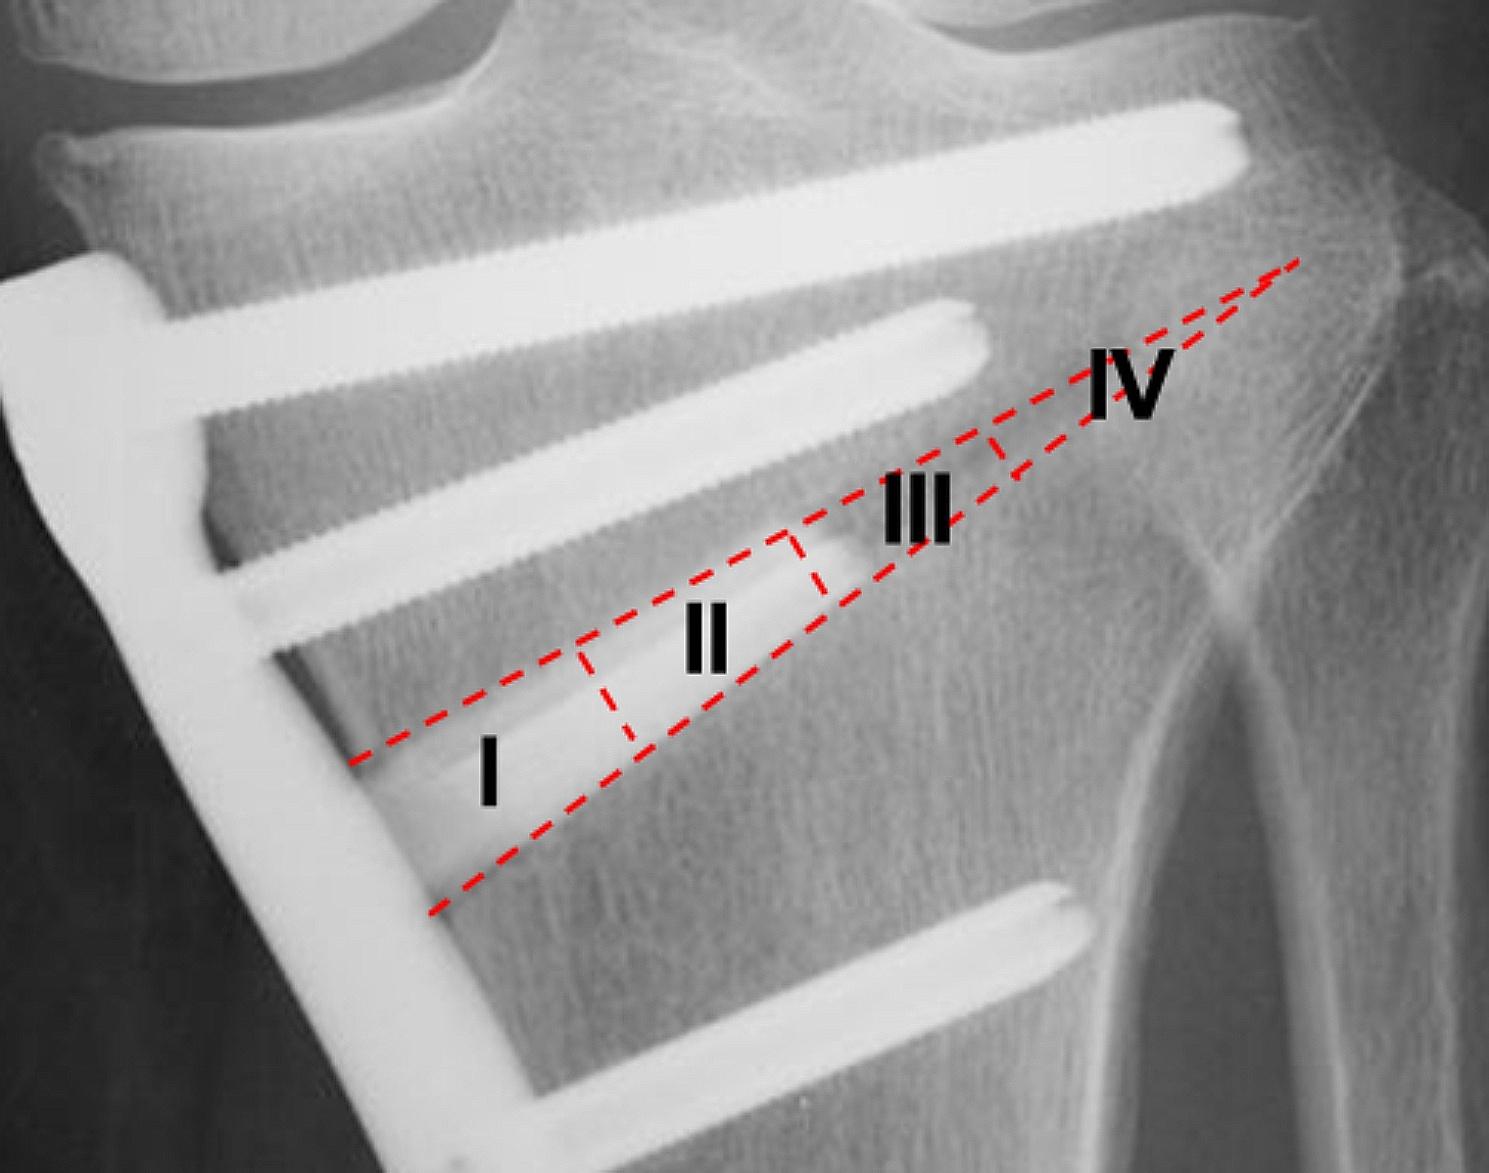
To evaluate the osteoconductivity of the implanted spacer, an elongated triangle which showed the wedge-shaped space created by open-wedge HTO was drawn on a radiograph. This elongated triangle was divided into four zones, Zone I (the most medial), II, III, and IV (the most lateral)
